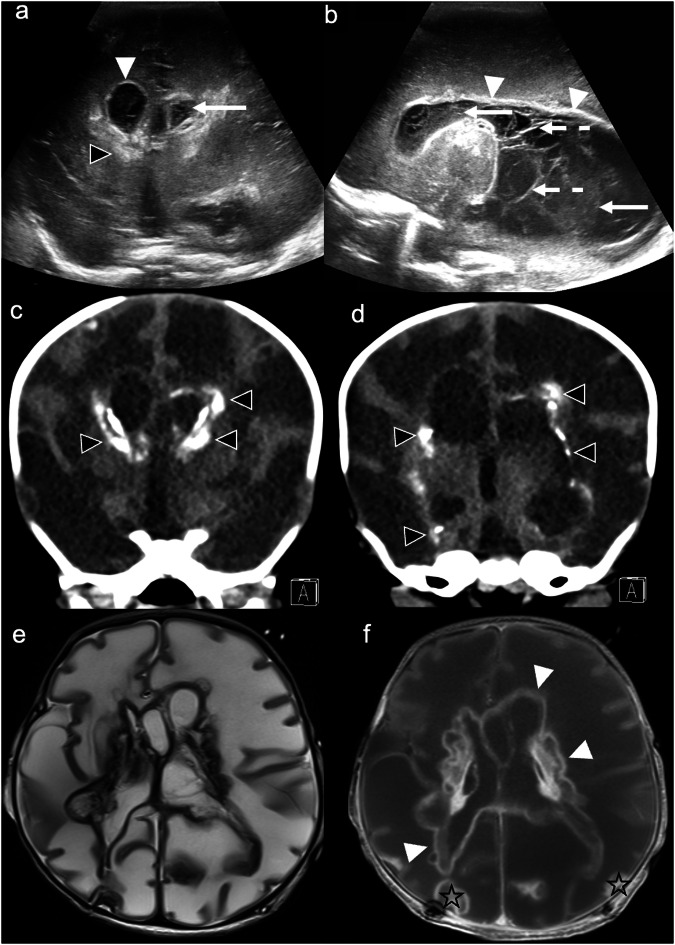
Congenital toxoplasmosis infection. Day 13 ultrasound in a term neonate with seizures and a bulging anterior fontanelle. a Coronal and b parasagittal images demonstrate severe ventriculitis with intraventricular debris (solid arrows), septations (dashed arrows), ventriculomegaly and ependymal thickening/increased echogenicity (white arrowheads); there is also periventricular echogenicity with posterior acoustic shadowing indicating calcification (black arrowhead), as well as diffuse brain swelling and diffusely increased parenchymal echogenicity with reduced grey-white differentiation. c, d Coronal CT images demonstrate coarse bilateral periventricular calcifications (black arrowheads). e Axial T2WI demonstrates diffuse parenchymal high signal and brain swelling, consistent with cerebritis. f Axial post-contrast T1WI demonstrates diffusely thickened, enhancing ependyma indicating ventriculitis (white arrowheads), along with bilateral parietal rim-enhancing lesions, consistent with abscesses (*)
